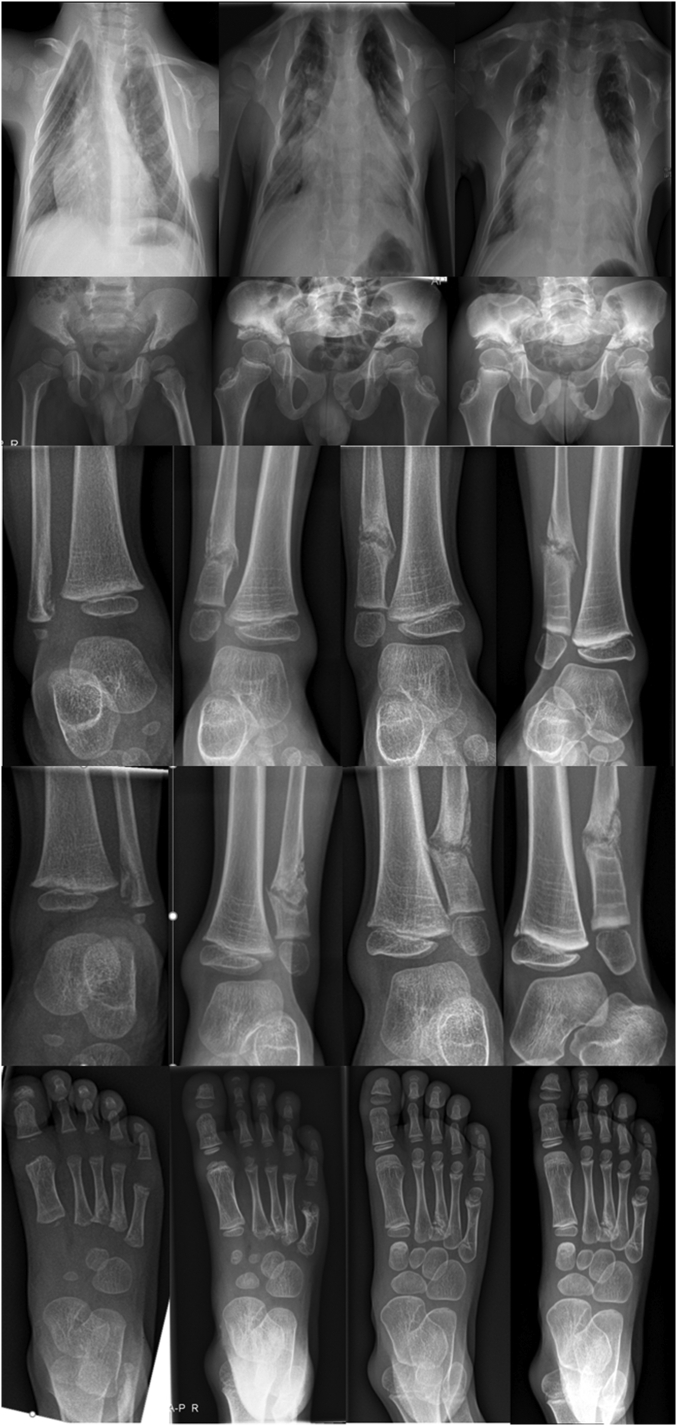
Radiologic findings at presentation and during follow-up. Upper row: Chest development at presentation (1 years 4 months) and during follow-up (3 years 5 months and 4 years 6 months). The chest radiographs shows relatively short down slanted ribs with proximal sclerotic irregularities with at multiple levels interruption of the ribs posteriorly. Over time the chest narrows. Also note the irregular aspect of the medial claviculae. Second row: AP pelvic radiograph at presentation, and 25 and 38 months after first presentation. Note the irregular and dysplastic acetabular development. The irregularities on the inside of the pelvic ring have normalized over time. Note growth resumption lines in the iliac bones and proximal femora as a result of bisphosphonate treatment. Third and fourth row: Radiographs of both ankles at presentation and during follow-up to age 7 years. Imaging shows the development of bilateral symmetric anomalies radiographically resembling enchondromas. As a result of growth the lesions ‘move away’ from the growth plate. Over time the lesions develop into a ‘pseudo-arthrosis’. Note growth resumption lines in the distal tibia and fibula as a result of bisphosphonate treatment. Fifth row: AP radiographs of the feet at presentation and during 5 years of follow-up. Figures show an irregular ossification of the tuft of the first digit of the left foot, irregular ossification of the distal metaphysis of the fifth digit of the right foot, and a well demarcated lucency at the base of the third metatarsal of the right foot. The fifth metatarsal of the right foot shows an irregular distal metaphysis and significant shortening.
